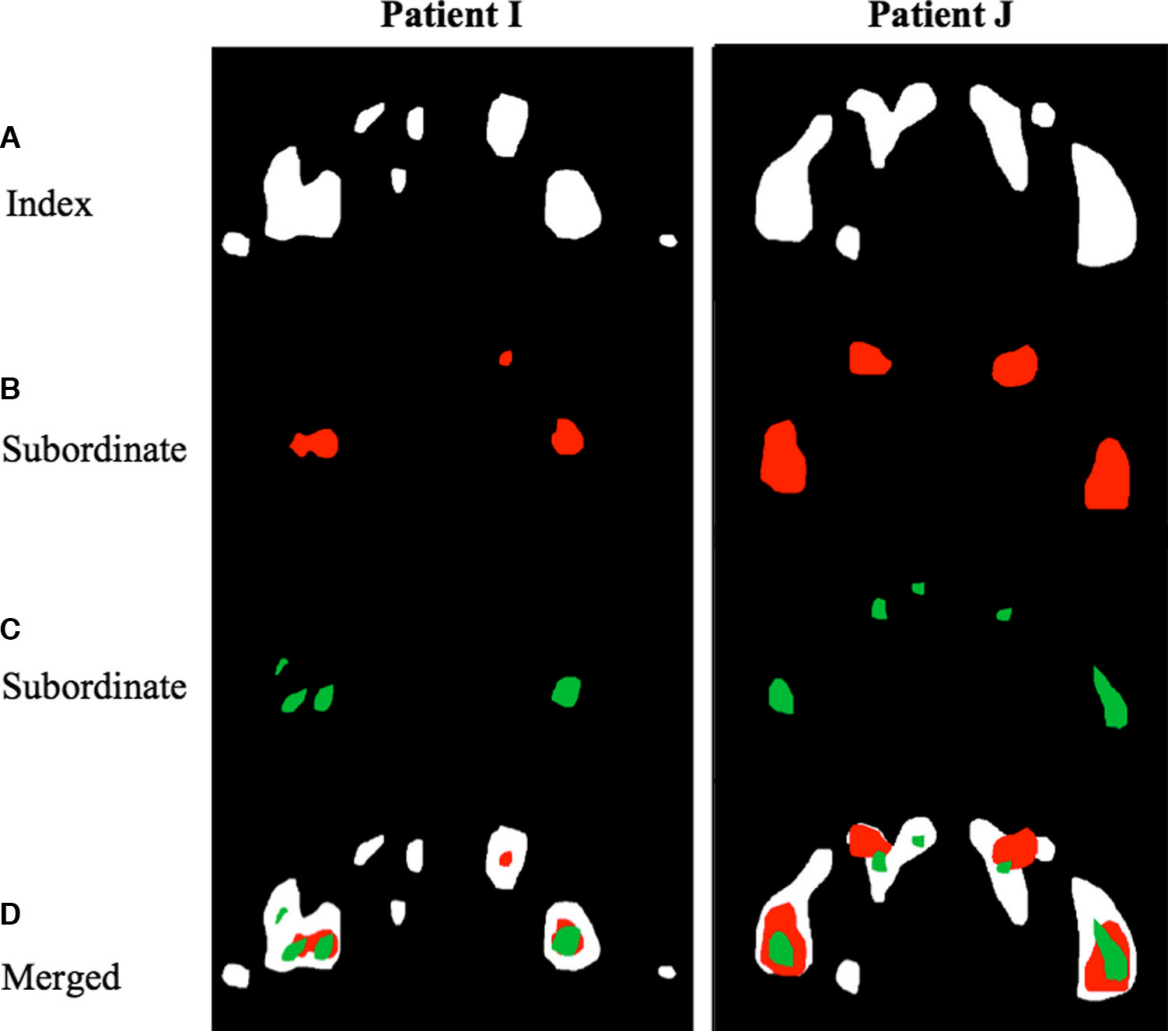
Serial PET/CT scans for two exemplar patients (I and J) showing segmented ^18^F‐FDG BAT from respective index scans (A), subordinate scans (B and C), with merged segmented images showing a high degree of visual colocalization (D).
